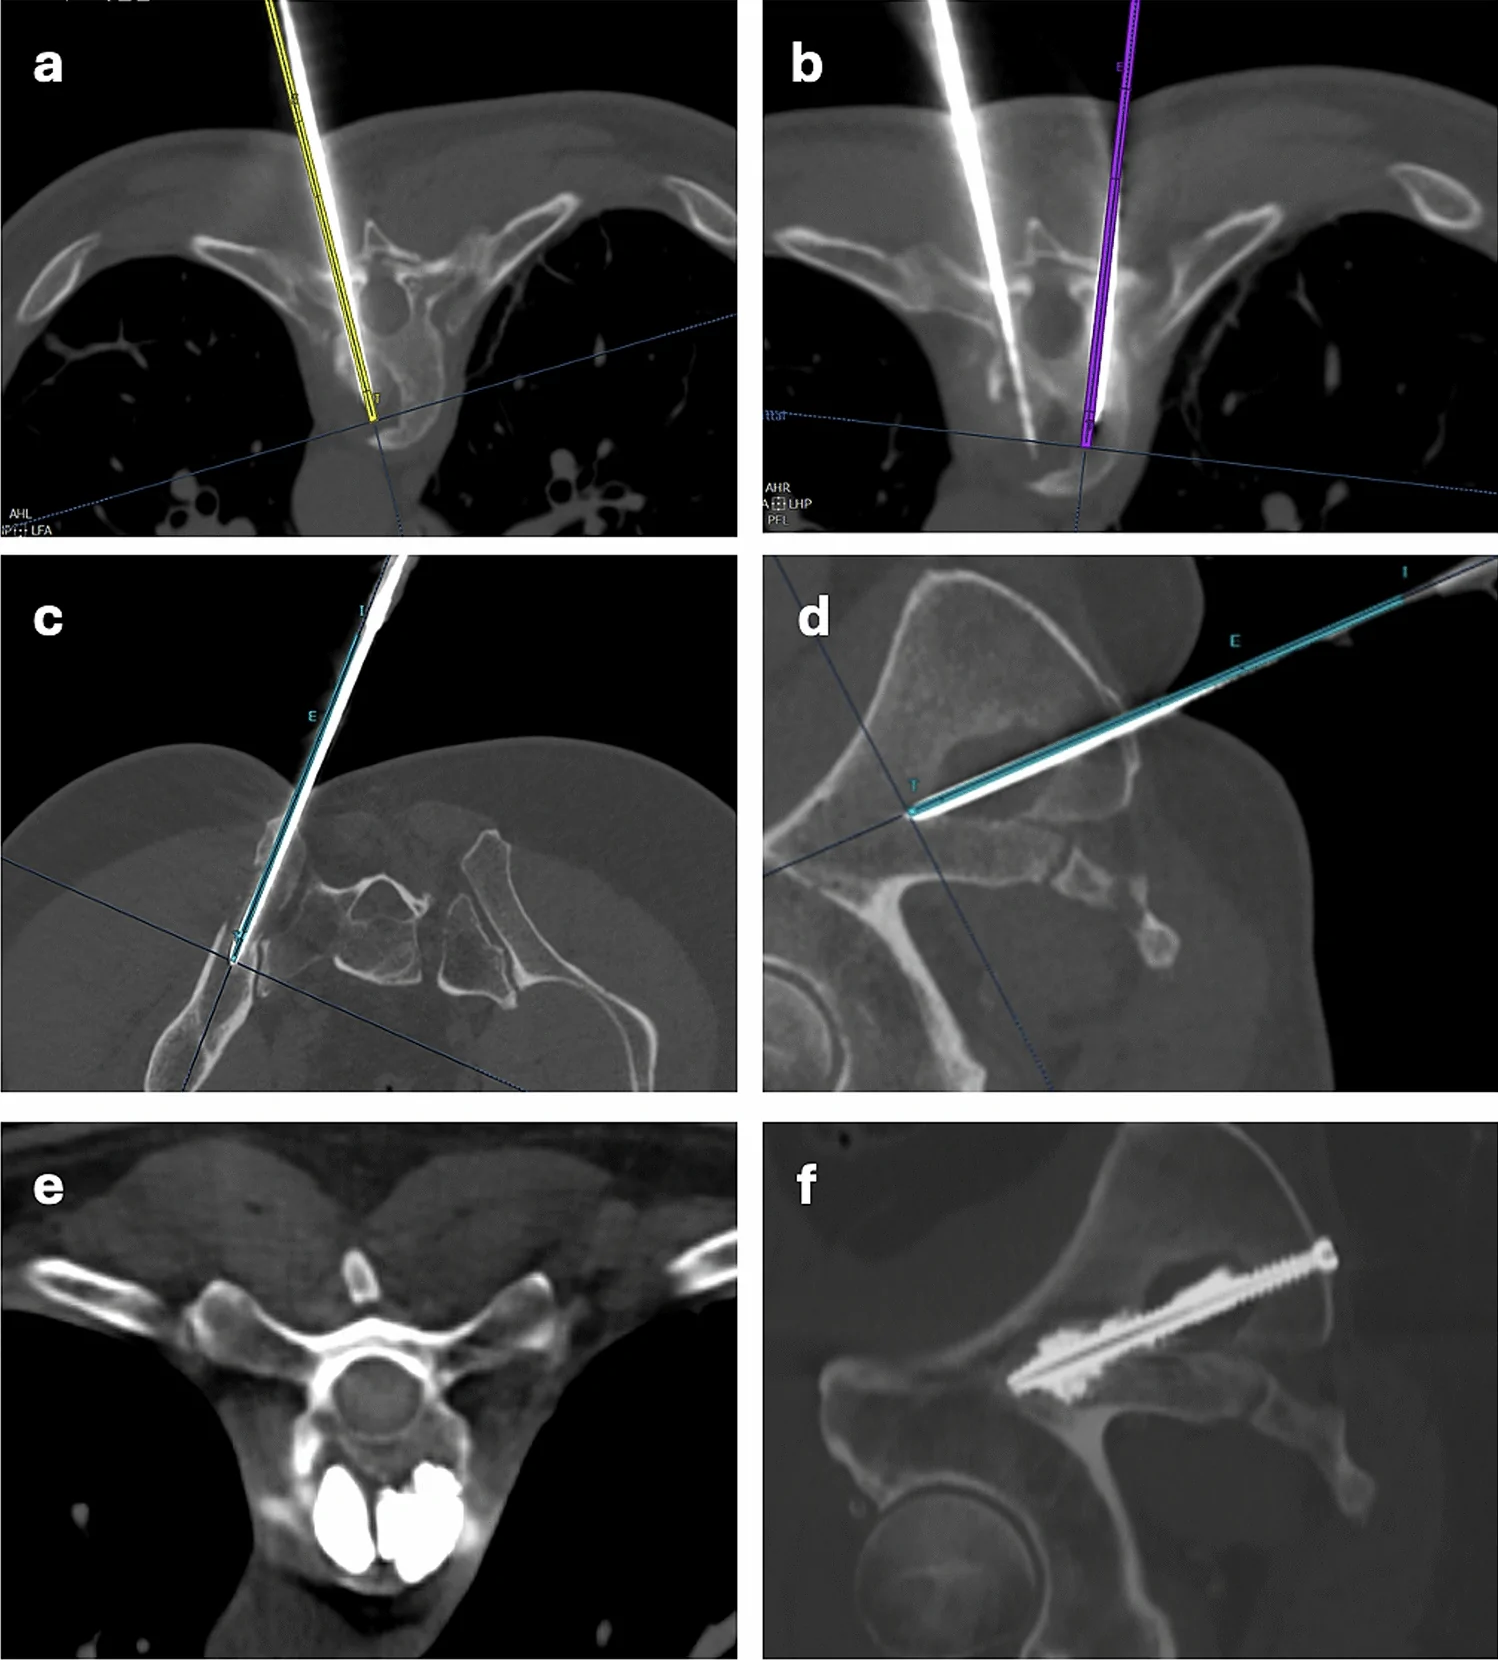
Representative case of combined spinal and pelvic consolidation. A 55-year-old patient with renal cell carcinoma receiving systemic therapy with cabozantinib presented with lytic bone metastases at risk of fracture involving the T8 vertebral body and the left iliac wing. The patient underwent T8 vertebroplasty and cement-augmented fixation of the left iliac wing. (a, b) Intraoperative axial CT images at T8 showing bilateral pedicular access with cementoplasty trocars aligned with the planned robotic trajectories (yellow and violet). (c, d) Intraoperative axial and oblique sagittal CT images of the pelvis showing trocar placement through the iliac lesion via a posterior approach, aligned with the planned trajectory (light blue). (e) Post-procedural axial CT image at T8 showing satisfactory cement distribution within the vertebral body. (f) Post-procedural oblique sagittal CT image of the pelvis showing satisfactory screw placement across the iliac lesion with distal cement anchoring
